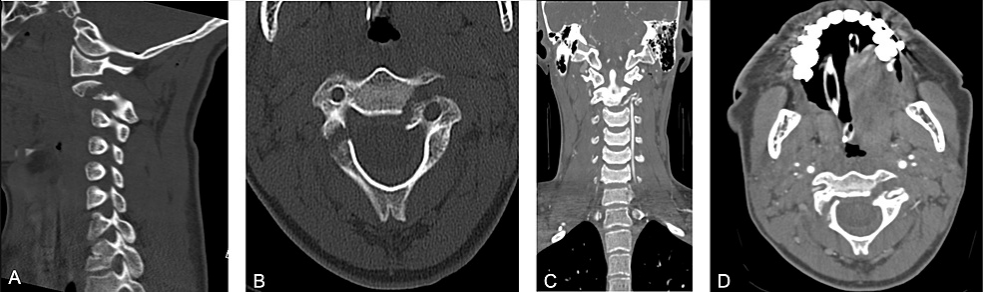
Pre-operative CT scan A, B. C2 vertebral body fracture involving the right pars and lamina, as well as a left laminar fracture involving the pedicle infiltrating the vertebral body and angulation of the dens. Also shown is C2 on C3 subluxation and anterior dislocation. C, D. Vascular imaging reveals dissection of the right vertebral body.
